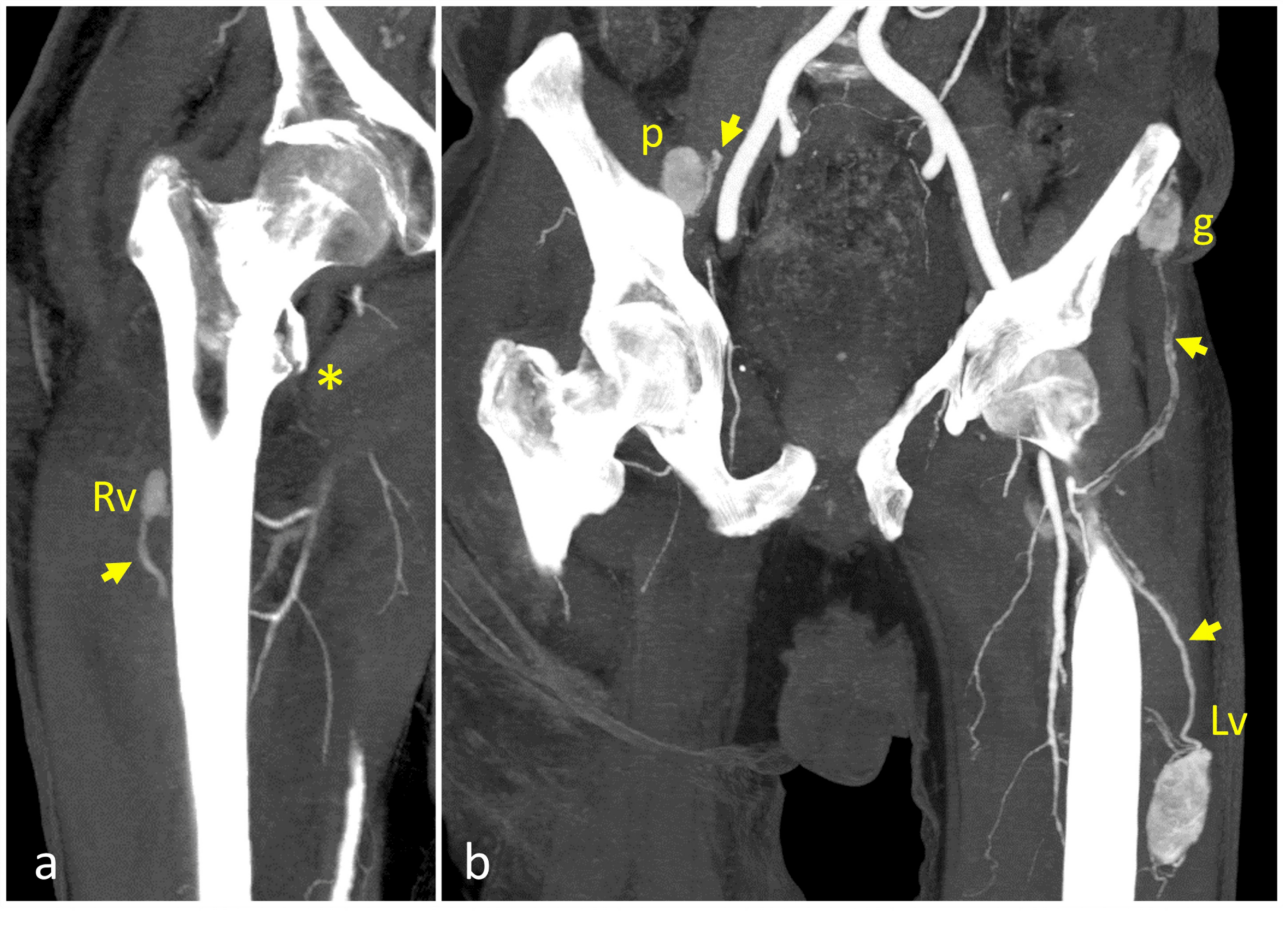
Arterial-phase CT with maximum intensity projection (MIP) of the pelvis and hips Image shows multiple hypervascular intramuscular masses with large feeding arteries and a right intertrochanteric fracture (*). Muscles highlighted include right vastus intermedius (Rv), right iliopsoas (p), left gluteus medius (g), and left vastus lateralis (Lv) with their corresponding feeding arteries (short arrows).
